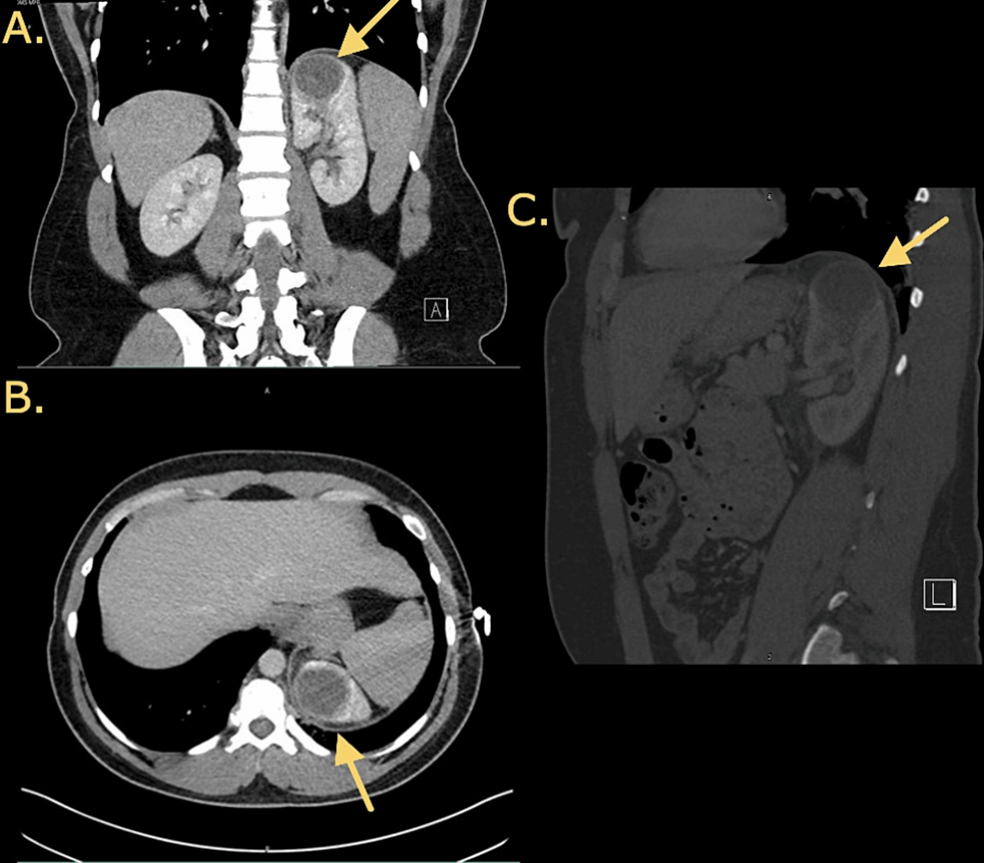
CT of the abdomen with contrast of the renal abscess showing a 4.1 x 4.1 x 3.8 cm rim-enhancing low-attenuation focus involving the upper pole of the left kidney. (A) Coronal view with the arrow indicating left renal abscess. (B) Axial view with the arrow indicating left renal abscess. (C) Sagittal view with the arrow indicating left renal abscess.
